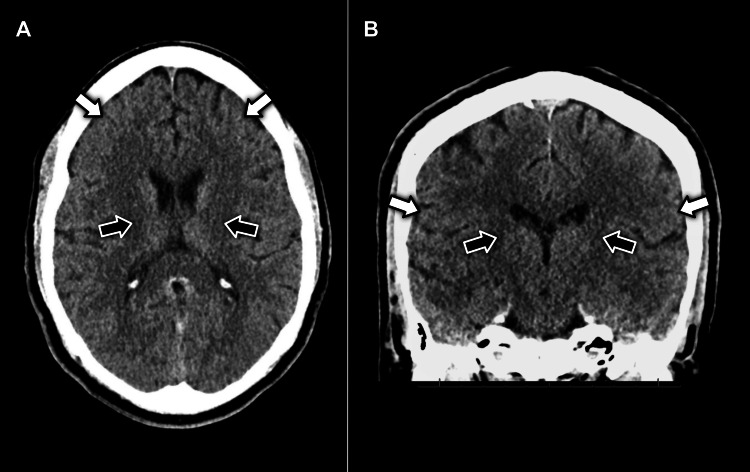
(A) axial and (B) coronal view CT imaging of the head without contrast displaying no evidence of pathology in the facial motor cortex (white arrows) or neuron pathways in the internal capsule (black arrows)
